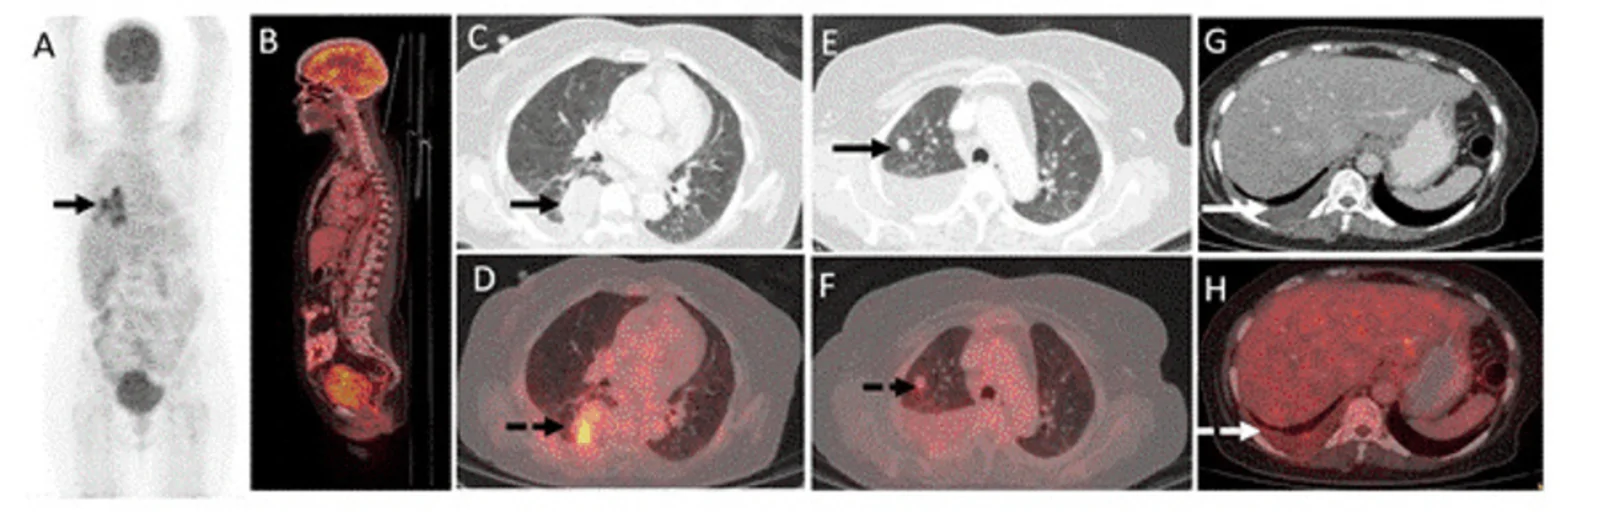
Pre-treatment FDG PET/CT demonstrating metastatic pulmonary and pleural disease Pre-treatment ^18F-FDG PET/CT images. (A) Maximum intensity projection (MIP) image demonstrating increased FDG uptake in the right hemithorax (black arrow). (B) Sagittal fused PET/CT image showing post-hysterectomy status without evidence of FDG-avid local residual or recurrent disease. (C, E) Axial CT images (black arrows) and (D, F) corresponding fused PET/CT images (black dotted arrows) demonstrating FDG-avid metastatic right lung nodules. (G) Axial CT image (white arrow) and (H) corresponding fused PET/CT image (white dotted arrow) showing FDG-avid right pleural effusion. FDG: fluorodeoxyglucose, PET/CT: positron emission tomography/computed tomography
